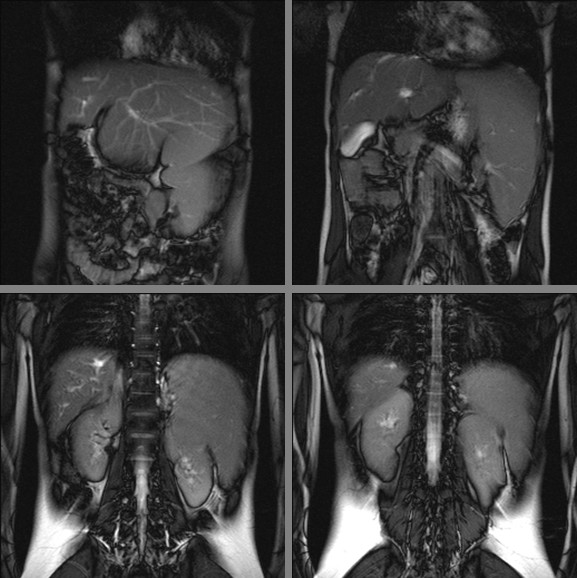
Abdomen MRI showing reduction of the volume of the right liver lobe, hypertrophy of the lateral segment of the left liver lobe and splenomegaly.
